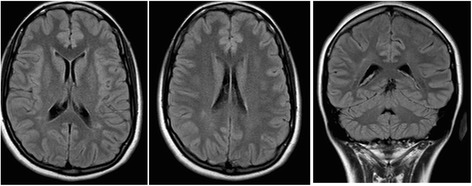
MRI scan images revealing aspecific peritrigonal white matter hyperintensities. The control MRI was performed after 6 months and 1 year and confirmed the small focal peritrigonal white matter hyperintensities in T2/FLAIR. Hypertension, heart valvular disease and other vasculophaties were excluded. Transcranial doppler US no detected the micro-embolic signals. Another MRI was performed after 2 years and the picture is unvaried
